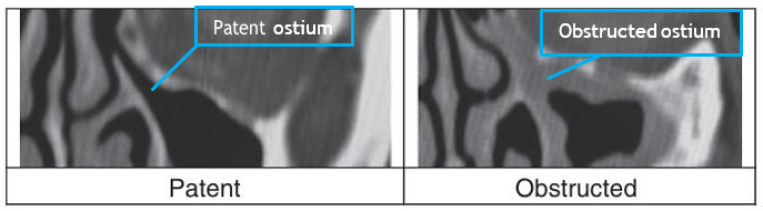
Coronal section of CBCT scans depicting the difference in appearance between a patent and obstructed ostium, adapted from Carmeli et al. [16].
